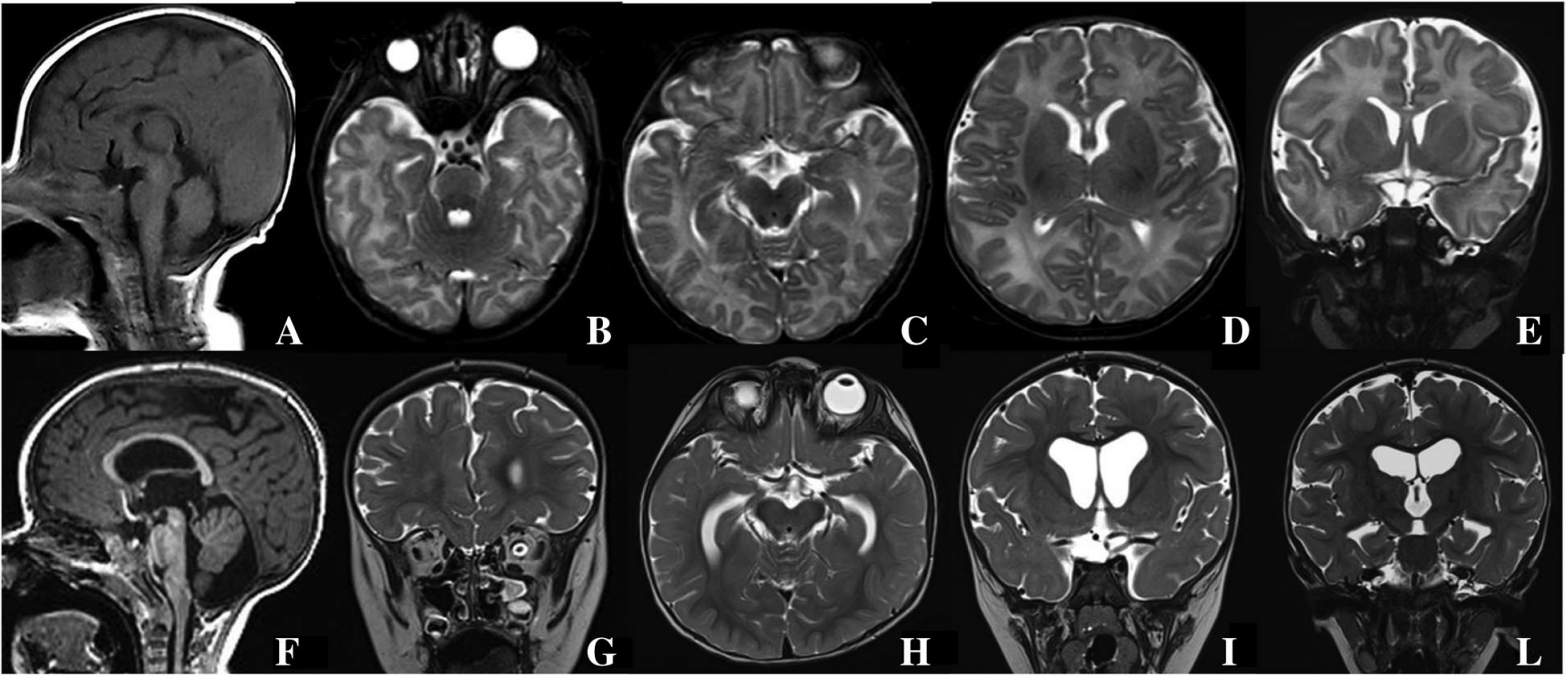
Brain MRI at birth and at the age of 18 months. Brain MRI at birth (a-e): sagittal T1-weighted spin echo (SE) (a), axial T2-weighted turbo spin echo (TSE) (b-d), coronal T2-weighted TSE (e). The examination shows right microphthalmia (b) and agenesis of the right optic nerve and hemi-chiasm (a-c), normal pituitary gland and stalk (a and e), small cranial posterior fossa with vertical and caudal tentorial implant, and a wider-than-normal IV ventricle due to cerebellar vermis hypoplasia (a). No molar tooth sign is evident at the midbrain level (c). Follow-up brain MRI at the age of 18 months (e-l): sagittal T1-weighted SE (e), coronal T2-weighted TSE (f, i, l) and axial T2-weighted TSE (h). The examination confirms the eyeball, optic nerve and posterior fossa findings, and clearly displays slight vermian dysmorphism and a wide communication between the IV ventricle and the basal cisterns (f), with regular superior cerebellar peduncles (f), corpus callosum hypoplasia (f), ventricular enlargement (i and l), incomplete hippocampal inversion (l) and pituitary gland hypoplasia (f and i)
